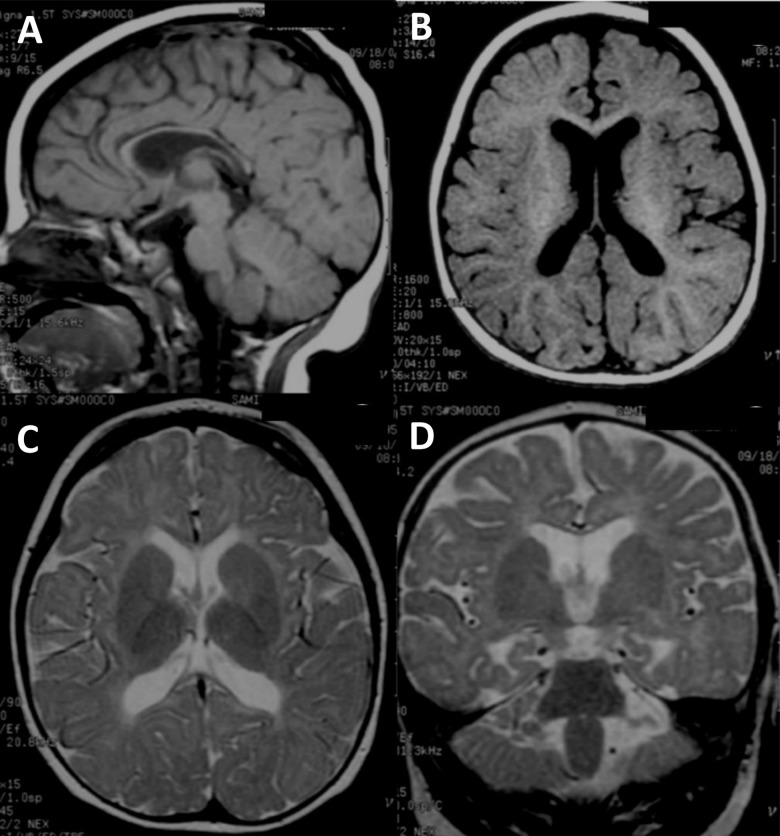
Brain MRI image of the ASD proband. (A–D) Brain MRI of the ASD proband at 10 months of age (II-2). (A) T1-weighted sagittal image shows fully formed but diffusely thin corpus callosum. The cerebellar vermis is normal. (B) T1-weighted axial image reveals normal gyral pattern of cerebral cortex and diminished cerebral white matter volume. (C) T2-weighted axial image demonstrates delayed myelination at anterior limbs of both internal capsules and diffuse white matter abnormalities seen as T2W hyperintensity at periventricular and subcortical white matter. (D) T2-weighted coronal image shows more obvious mild cerebral atrophy seen as enlarged CSF space and prominent temporal horns of both lateral ventricles. Diffuse white matter abnormalities at periventricular and subcortical white matter are noted.
